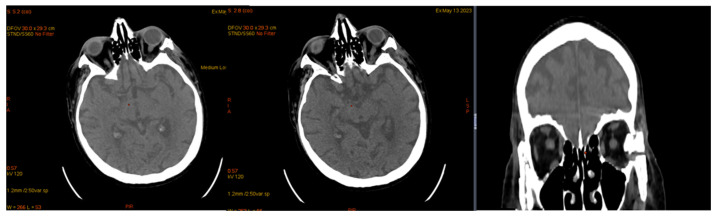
Cerebral CT, May 2023. The bilateral intraorbital tissue masses are no longer evident compared to previous examinations, and there are no areas of ischemia or meningoencephalic infiltration.
